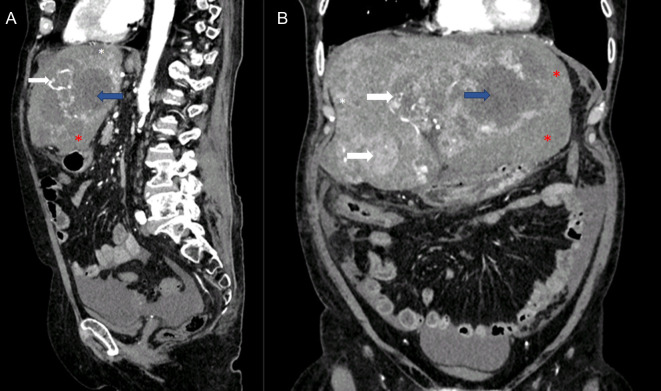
Abdominal frontal (A) and sagittal (B) CT images showing recurrent HCC (white arrow), liver hematoma (red arrow) and multiple liver abscesses (blue arrow).
